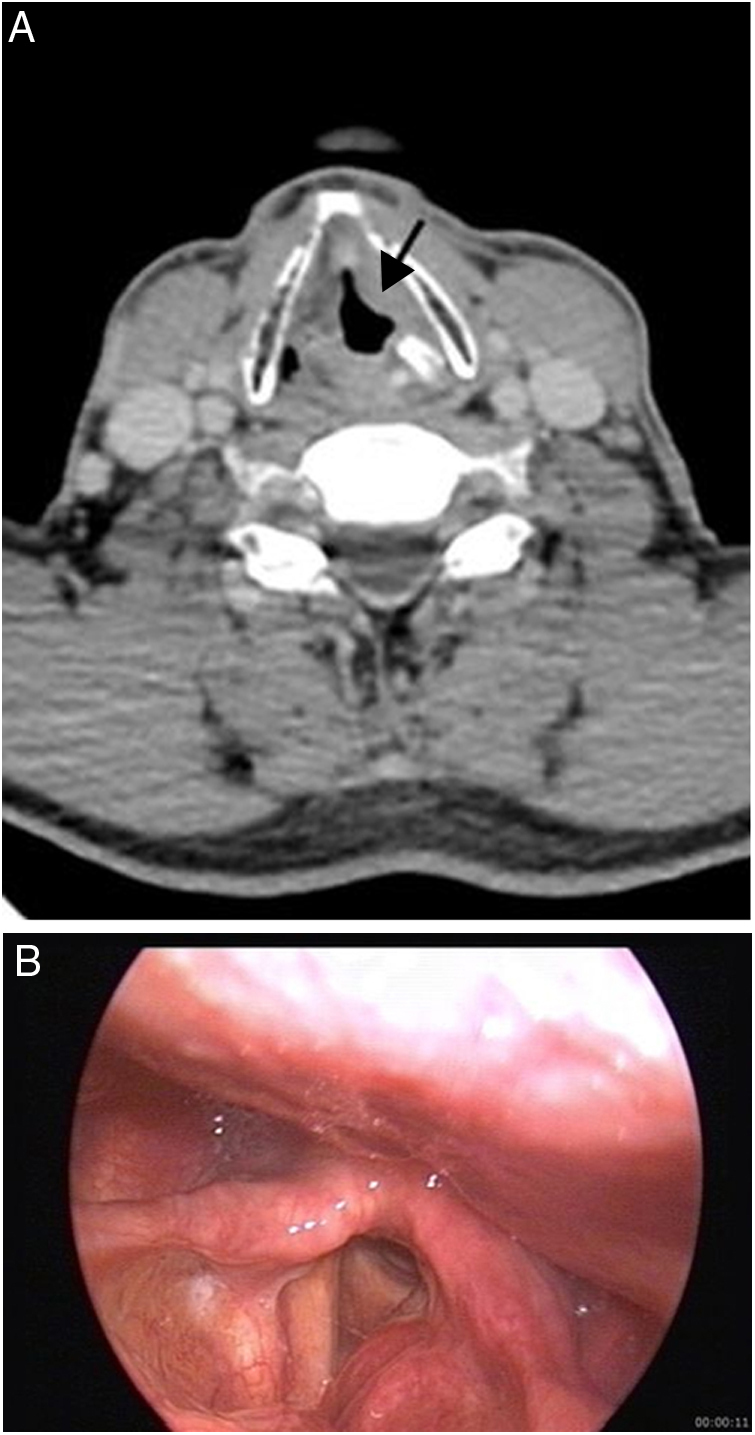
(A and B) 61 year-old man with pulmonary and laryngeal tuberculosis. Contrast enhanced CT image (A) shows a mass on the left band ventricle (arrow) and left arytenoid cartilage sclerosis. Endoscopic image (B) demonstrates a mass on the left band ventricle protruding on the left vocal cord.
